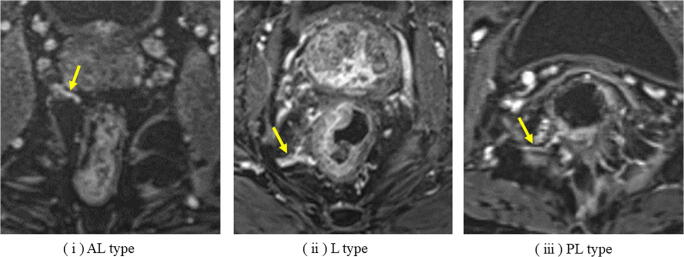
Three types of middle rectal artery detected by contrast-enhanced MRI axial slices. (i) AL type, antero-lateral type; (ii) L type, lateral type; (iii) PL type, postero-lateral type
